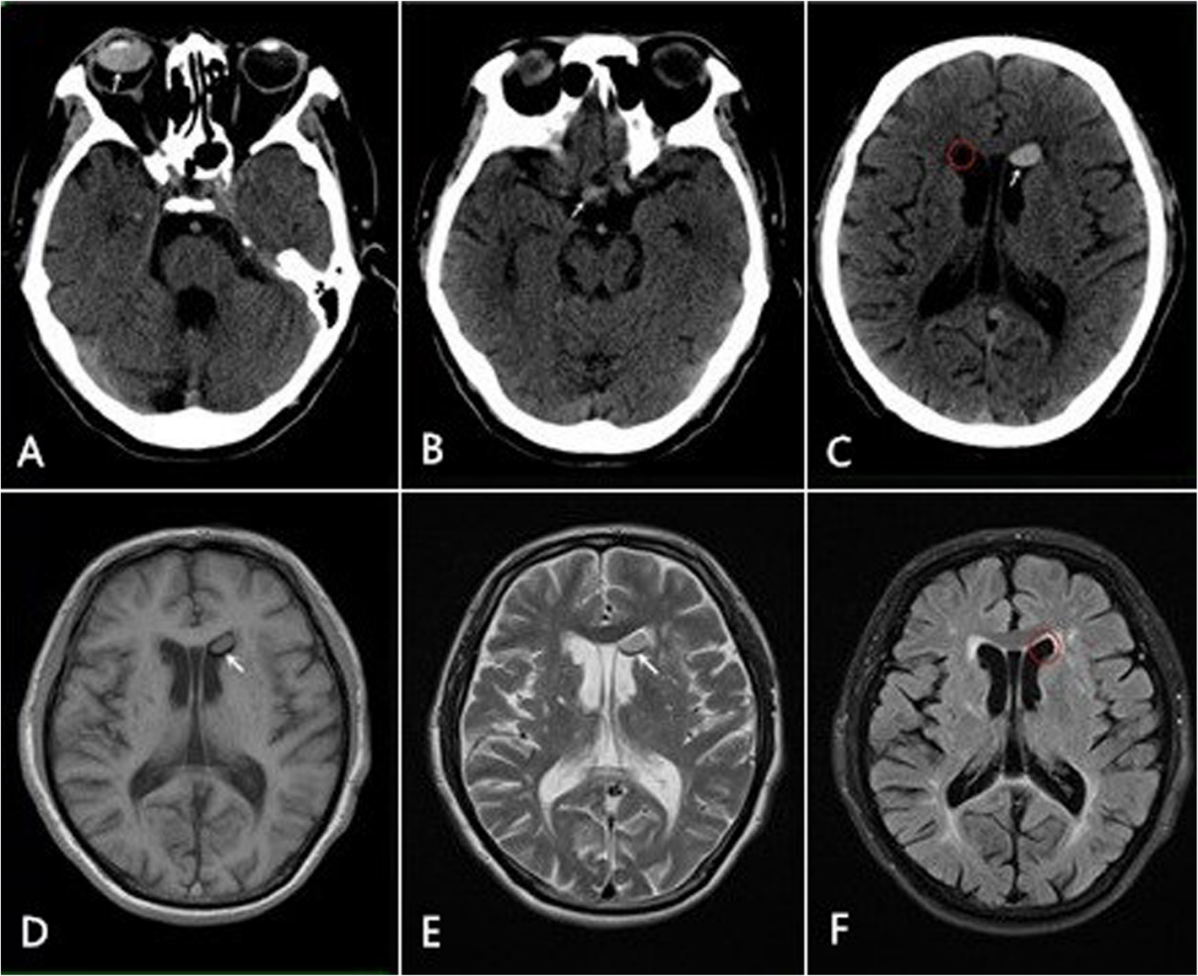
The patient receives CT and MRI examinations in July 2014. a~b, the high or slightly high density shadow on the right side of the right eyeball and the right suprasellar cistern (ww 60, wl 100)(arrow); c, the high-density shadow on the left ventricular anterior horn (arrow), disappearance of the high-density shadow on the right ventricular anterior horn (red circle) (ww/wl same as A); d~e, lesions with slightly high signal intensities on T1WI and T2WI, and chemical shift artifact on the edge of lesion (arrow); f, disappearance of the slightly high signal intensity on T2WI_tirm_dark-fluid (red circle)
